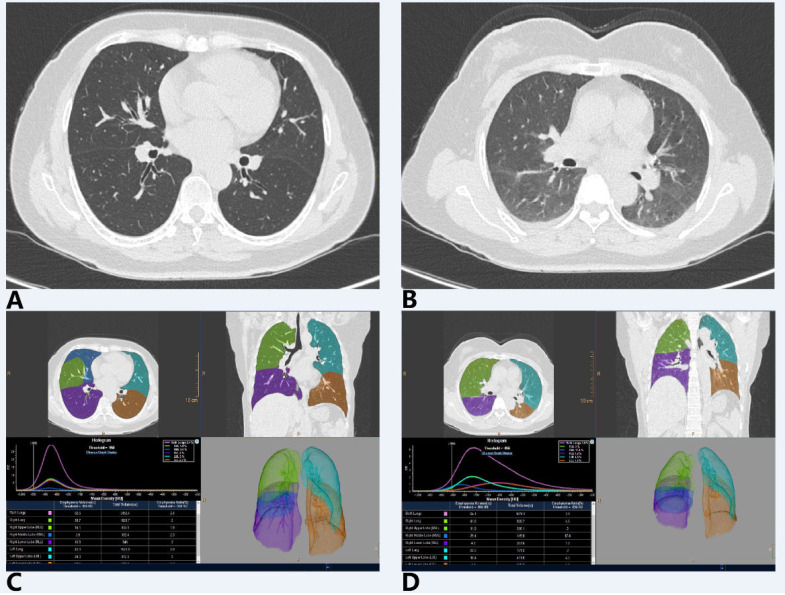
Paired inspiratory–expiratory chest CT image and postprocessing interface. (A) Chest CT image at deep-inspiratory phase; (B) Chest CT image at deep-expiratory phase; (C, D) Postprocessing and quantitative analysis on inspiratory and expiratory chest CT images.
